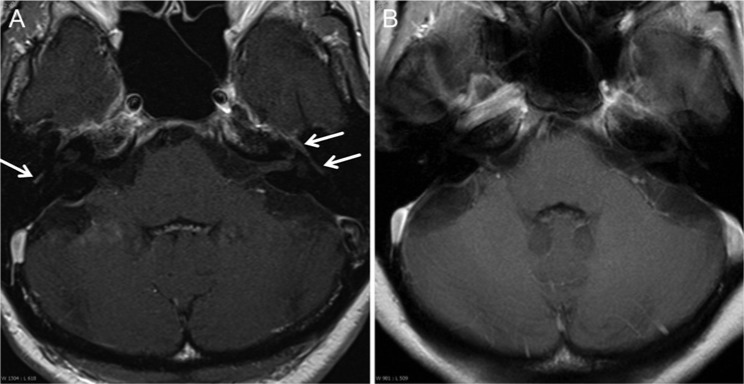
(A) MRI head: axial T1 weighted with contrast agent (August 2013): pathological enhancement of N. facialis on both sides in tympanal segment (arrows), expansion as far as Foramen stylomastoideum (not shown). (B) MRI head after treatment: axial T1 weighted with contrast agent (September 2013): enhancement of N. facialis in the tympanal segment is no longer detectable.
